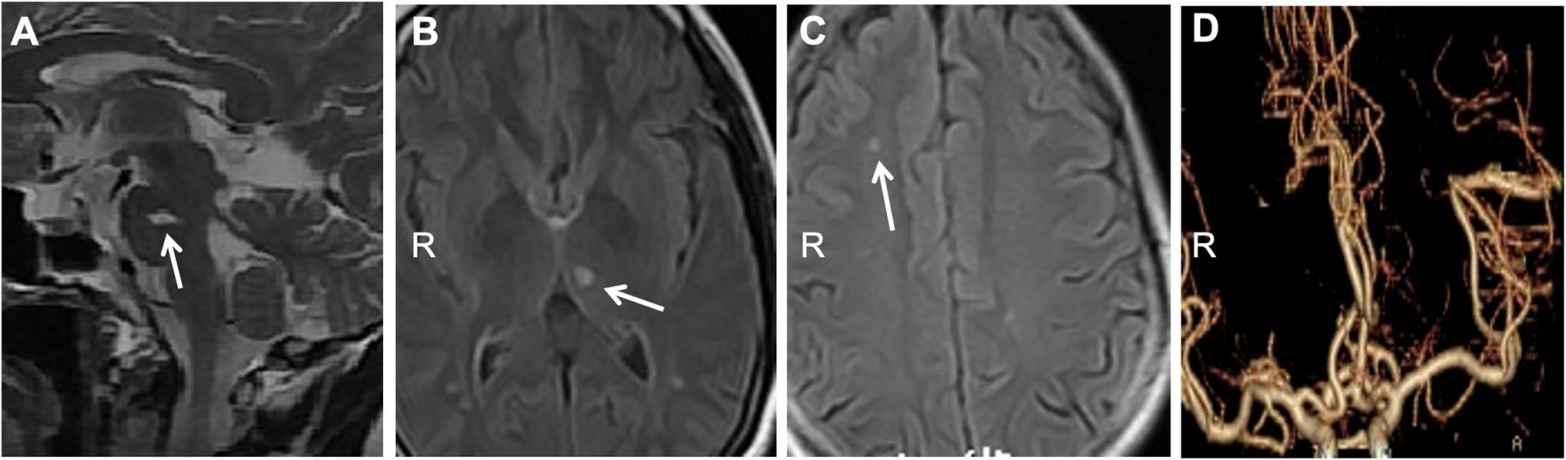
Initial MRI of the index case (III10). MRI brain for minor trauma workup showed abnormal hyperintensity signals in the pons (a, T2-weighted), the left thalamus (b, FLAIR) and subcortical white matter of the right frontal lobe (c, FLAIR). MR angiography of the brain (d) was normal
